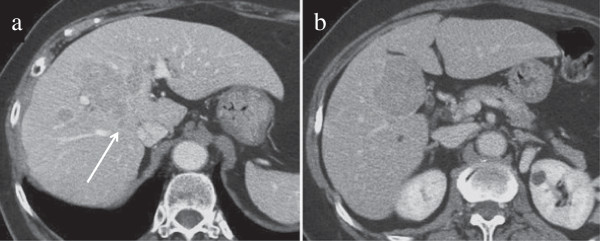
Computed tomography revealed a tumor located mainly at Couinaud’s segments 4, 5, and 8, and this tumor extended to the paracaval portion of the caudate lobe in the liver (arrow).
